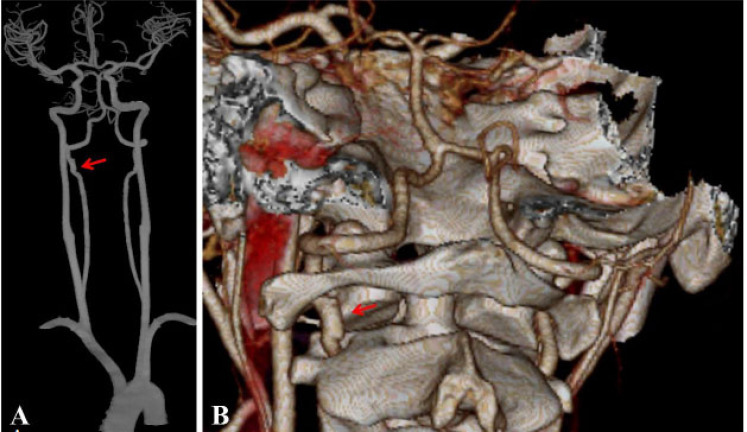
Cervical CTA. (A-B) Possible dissecting aneurysm of the right VA at the V3 segment.
